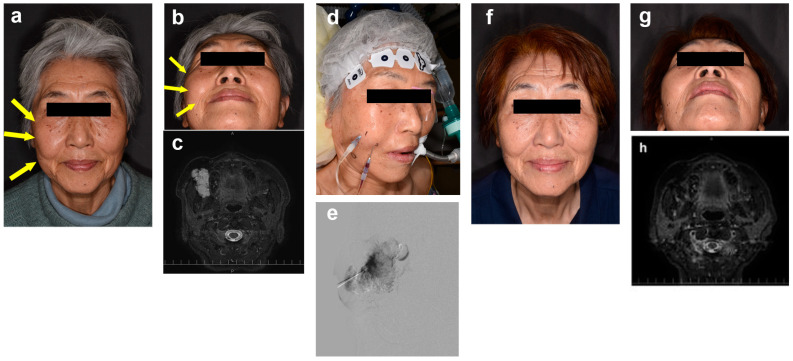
A 78-year-old female with a painful intramuscular venous malformation (VM) in the right buccal region. ((a,b), yellow arrows) Pre-sclerotherapy photographs (frontal and low-angle views). The yellow arrows indicate the lesion. (c) Pretreatment STIR MRI showing the lesion volume measured independently by two radiologists as 20.5 cm^3^ and 19.4 cm^3^ (mean: 20.0 cm^3^). (d,e) Photographs during sclerotherapy. Following the established protocol, 8.2 mL of 5% ethanolamine oleate (EO) was injected percutaneously under digital subtraction angiography (DSA) via direct percutaneous puncture. (f,g) Photographs 3 months after sclerotherapy (frontal and elevated-angle views). (h) Post-treatment STIR MRI showing lesion volumes measured as 6.4 cm^3^ and 6.3 cm^3^ (mean: 6.4 cm^3^), corresponding to a 68.2% reduction.
